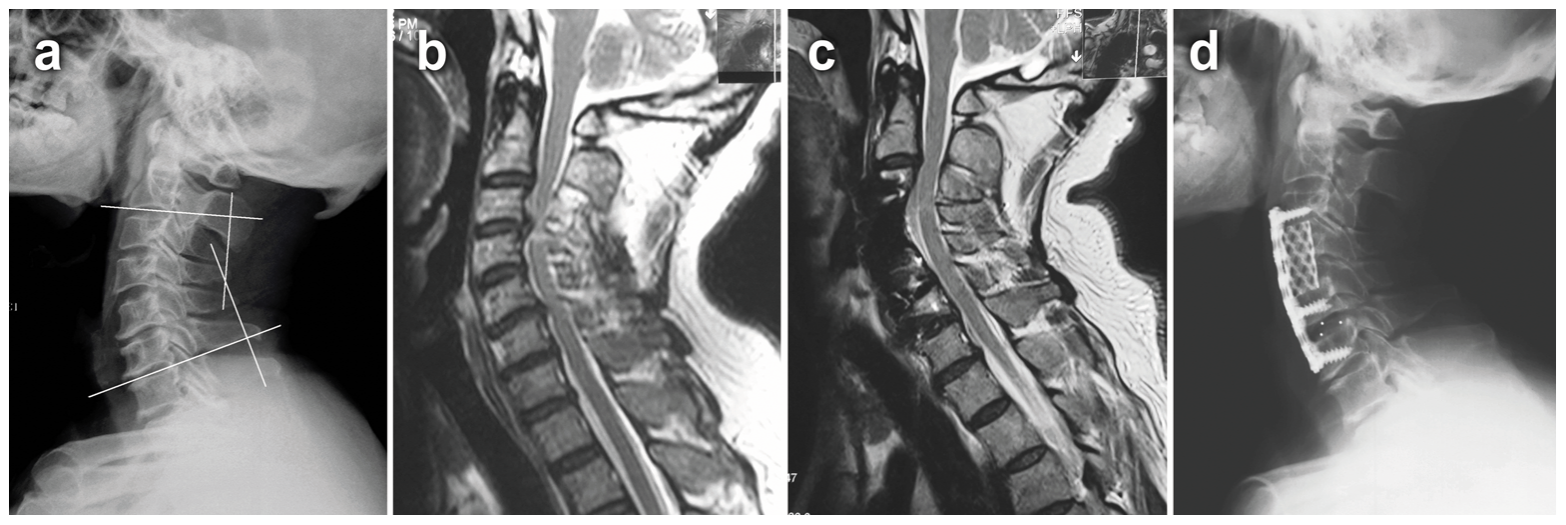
A 65-year-old male developed numbness in his two hands and weakness in his four extremities for 2 years. Preoperative imaging studies showed that the spinal cord compressed at C3–C6. He was performed with 3-level ACHDF. After operation, his JOA scores improved from 7 preoperation to 13 postoperation. a Preoperative lateral X-ray. The segmental lordosis of C2–C7 was defined as the angle formed by the lower endplate of C2 vertebral body and the upper endplate of C7 vertebral body. b Preoperative MRI. c 2-year postoperative MRI. d 2-year postoperative lateral X-ray.
